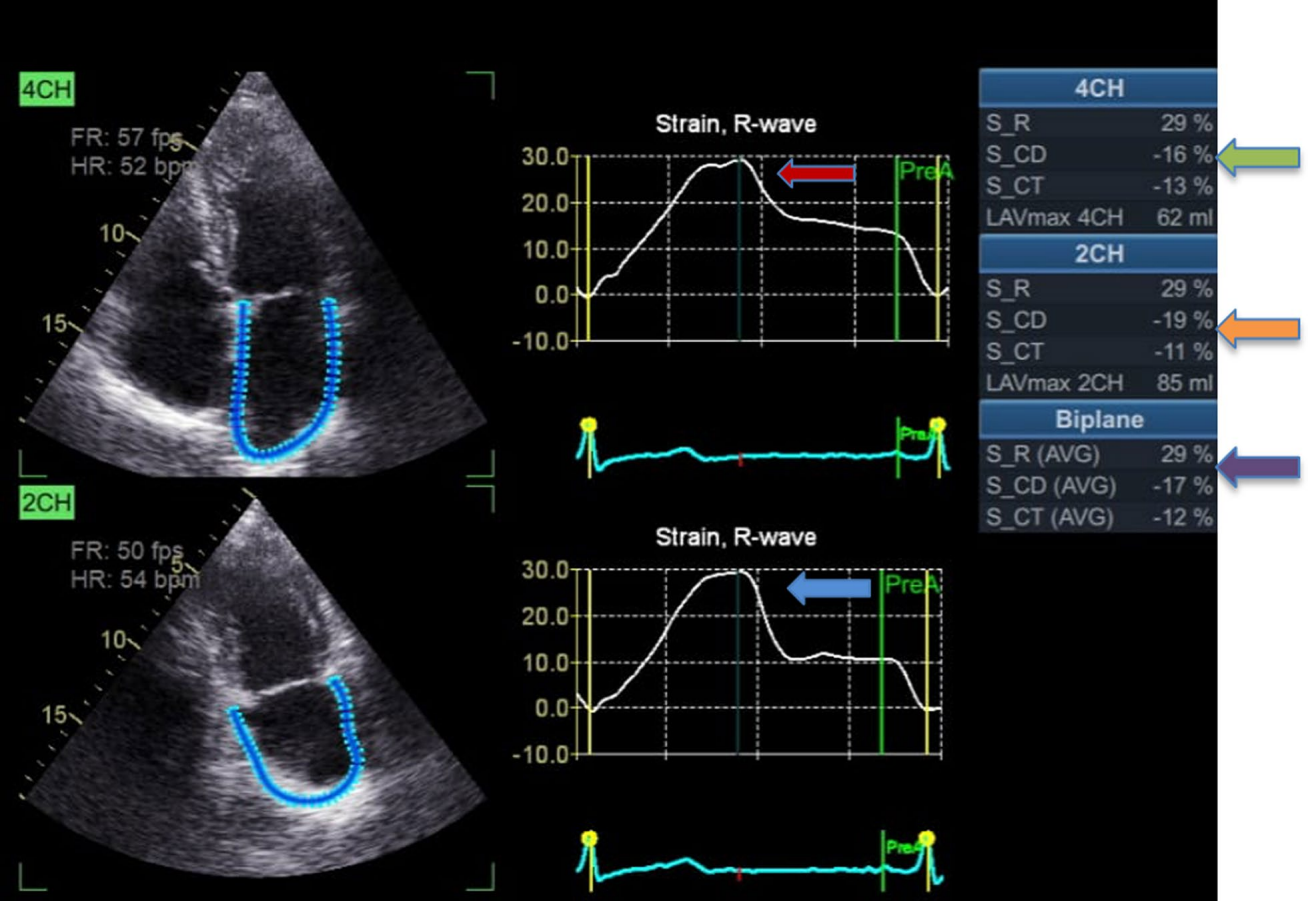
Measures of LA strain by speckle tracking. Red and blue arrows denote peak reservoir strains for the 4- and 2-chamber echocardiographic views, respectively. Green and orange arrows represent the automatic results for the reservoir, conduit, and contraction measures in the 4- and 2-chamber views, respectively. The purple arrow highlights the mean values for the two views
